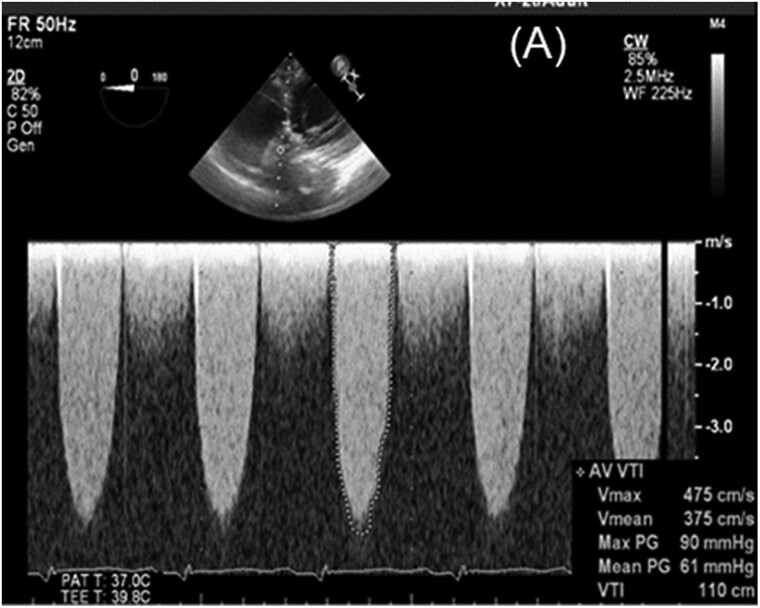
Trans-oesophageal echocardiogram demonstrating severe aortic obstruction.
